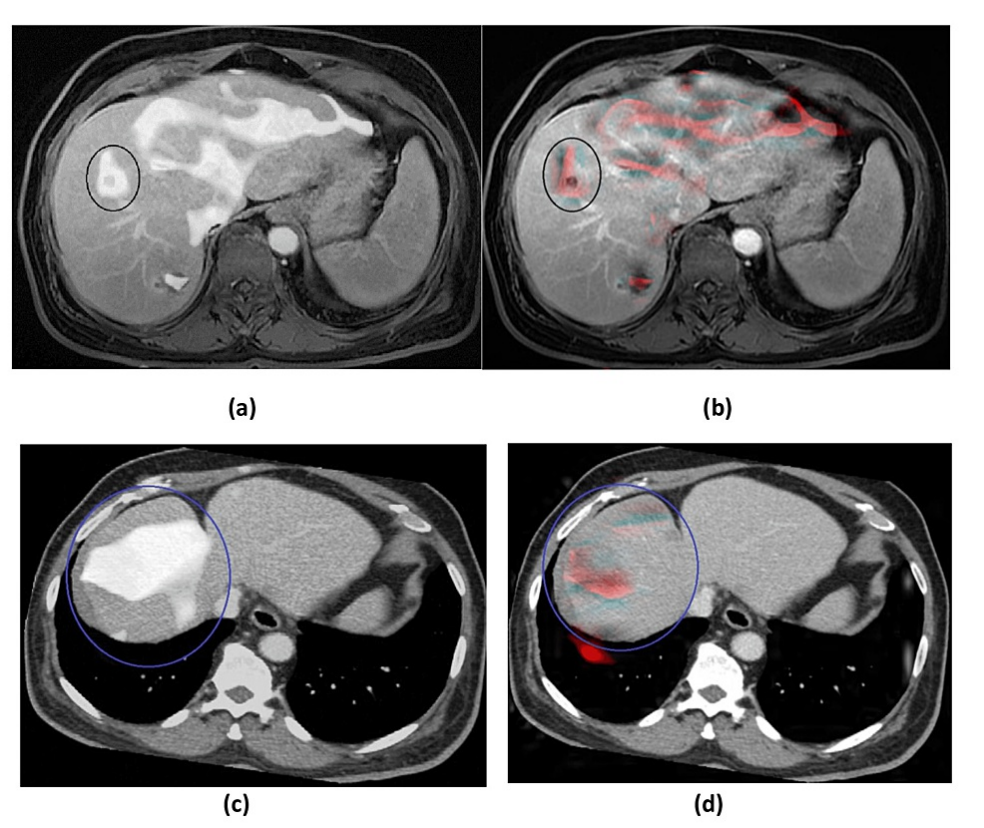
Comparison between the proposed method and DWT fusion. (a) Proposed method (MRI-MRE fusion), (b) DWT (MRI-MRE fusion), (c) Proposed method (CT-MRE fusion), (d) DWT (CT-MRE fusion). CT = computed tomography; MRI = magnetic resonance imaging; MRE = magnetic resonance elastography; DWT = discrete wavelet transform
